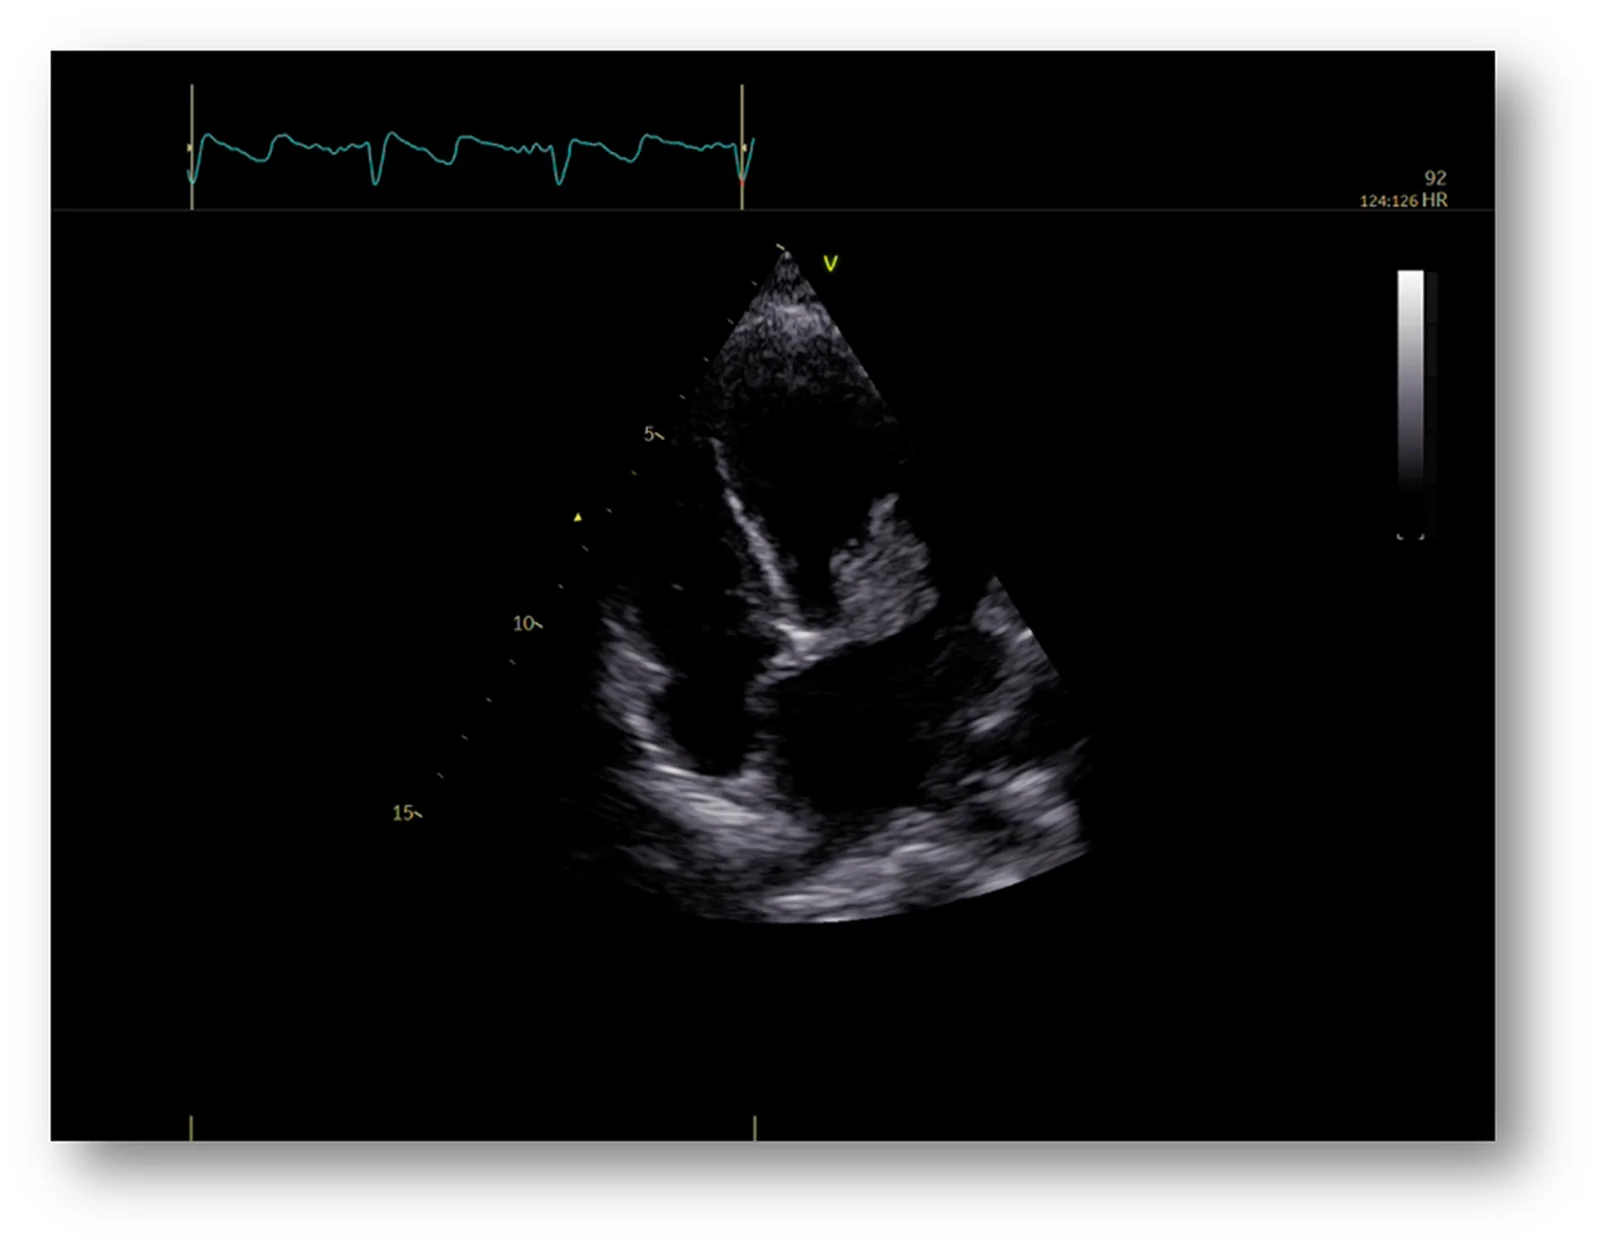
Apical four-chamber view demonstrating large vegetation on anterior mitral valve leaflet (Patient 1).
